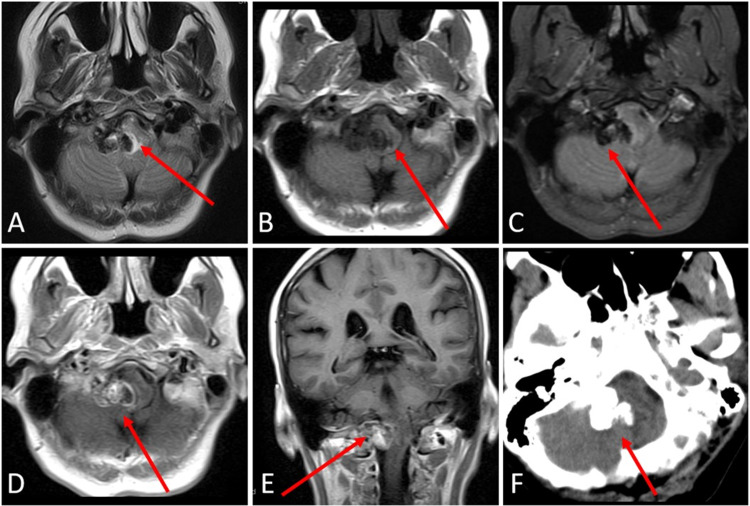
Preoperative imaging of a right posterior fossa calcifying pseudoneoplasm of the neuraxis (CAPNON). Axial T2 (A), T1 (B), and GRE (C)-weighted MRI of the brain demonstrates a predominantly T1 and T2 hypointense extra-axial mass centered in the posterior fossa on the right at the level of the lateral cerebellomedullary cistern. There is mass effect and T2 hyperintensity in the medulla compatible with vasogenic edema (arrow, A). There is blooming artifact on GRE (C) compatible with calcification. Axial (D) and coronal (E) post contrast T1-weighted images demonstrate heterogenous enhancement. Axial non-contrast CT head demonstrates a predominantly calcified mass centered in the posterior fossa in the right lateral cerebellomedullary cistern (F). GRE: gradient recalled echo
